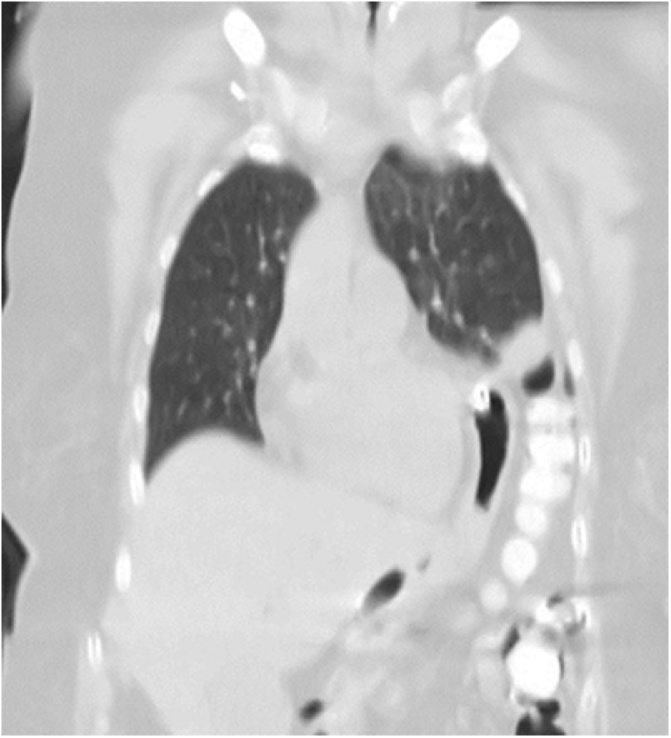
Whole-body oral-contrast CT scan (coronal view with pulmonary window).
